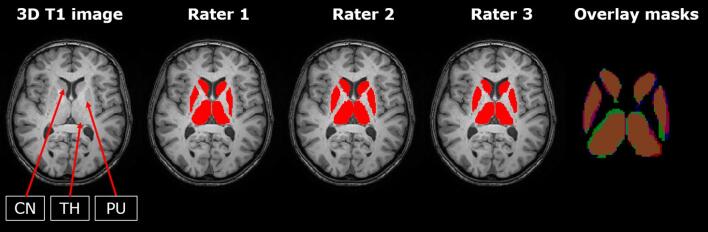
Overview of manual delineations of the 3 raters and their overlap. From left to right: Axial 3D T1-weighted MRI slice with segmentations, 2D view of manual reference of rater 1 to 3 and 2D view of overlap of raters with green, blue and red one rater, purple and pink two and orange three raters. (For interpretation of the references to colour in this figure legend, the reader is referred to the web version of this article.) Abbreviations: CN = caudate nucleus; TH = thalamus; PU = putamen.
